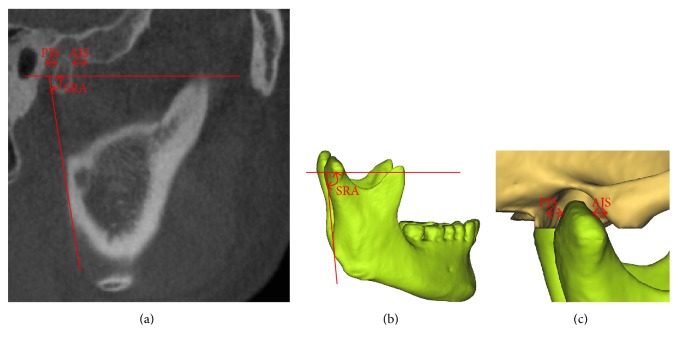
Measurements of the SRA, PJS, and AJS in the sagittal view: (a) on the CBCT images and (b and c) in the 3D models. SRA: sagittal ramus angle; AJS: anterior joint space; PJS: posterior joint space.
